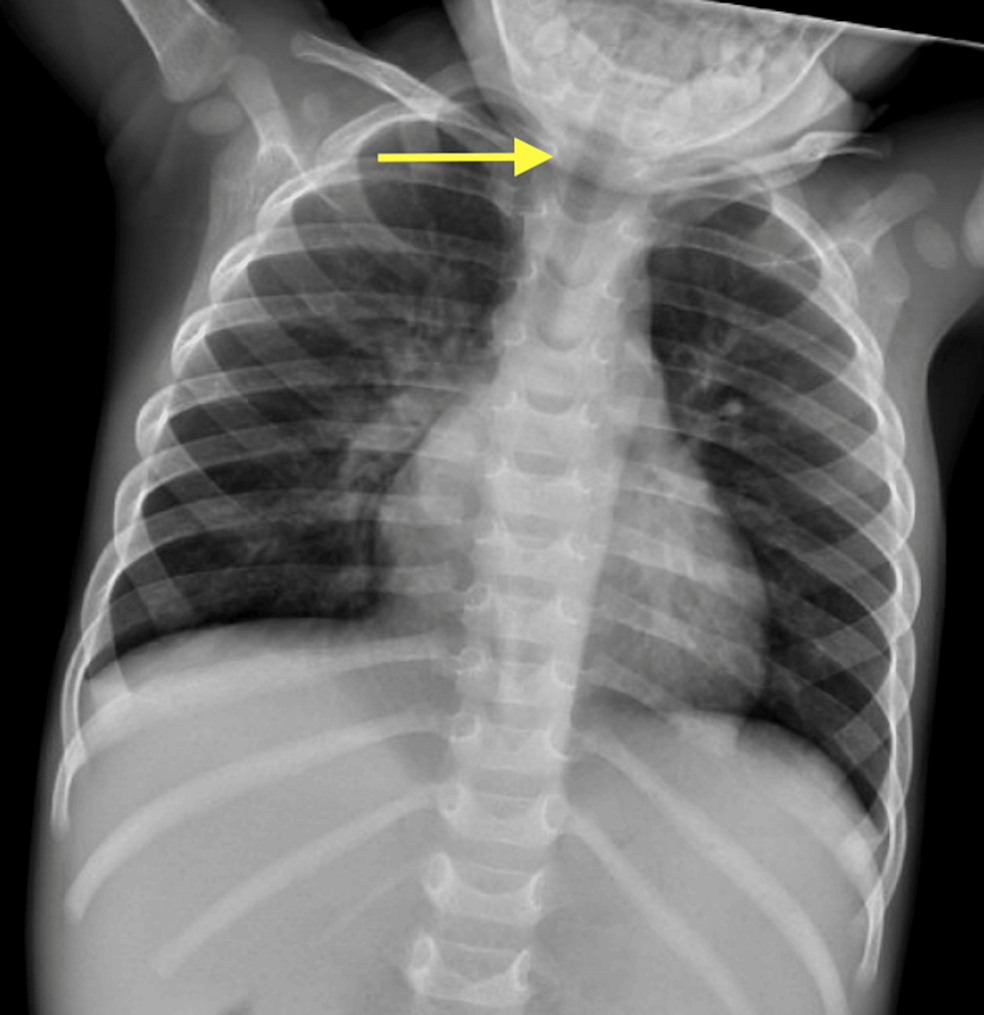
Chest radiograph on day of discharge from initial pediatric intensive care unit (PICU) admission showing clinical improvement of stenosis
